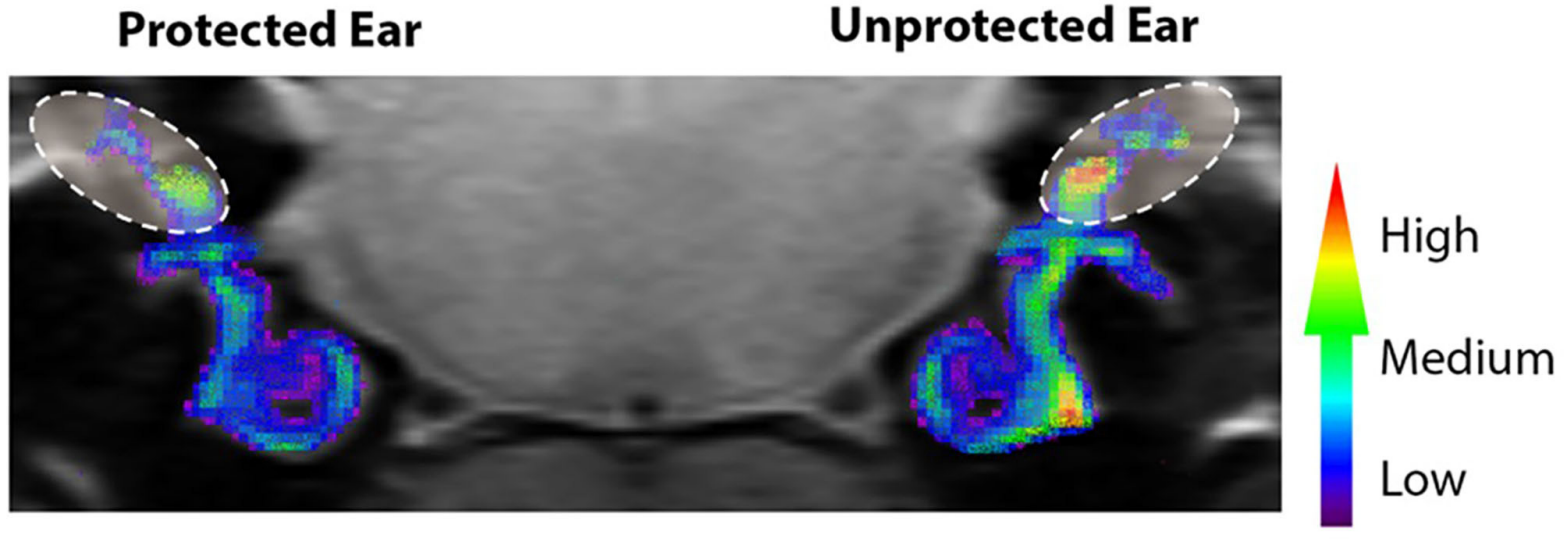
Noise-evoked inner ear oxidative stress measured *in vivo* with QUEST MRI. R1 (1/T1) maps were generated and used to compare protected (plugged ear) and unprotected vestibular end organs and cochleae. Scale bar is a graded colorimetric representation of R1 values. High values are represented as a gradient from red to yellow and low values are represented from blue to purple. Vestibular regions–area within ovals with dashed lines. The R1 values were collected *in vivo* from 400 μm MRI scans. Scans through the cochlear and vestibular ducts were sampled and analyzed from caudal to rostral. Therefore, the coronal image in Figure 4, provides views of the regions of interest from caudal to rostral (e.g., the right cochlea is viewed on the right side of the image). Adapted from (22).
